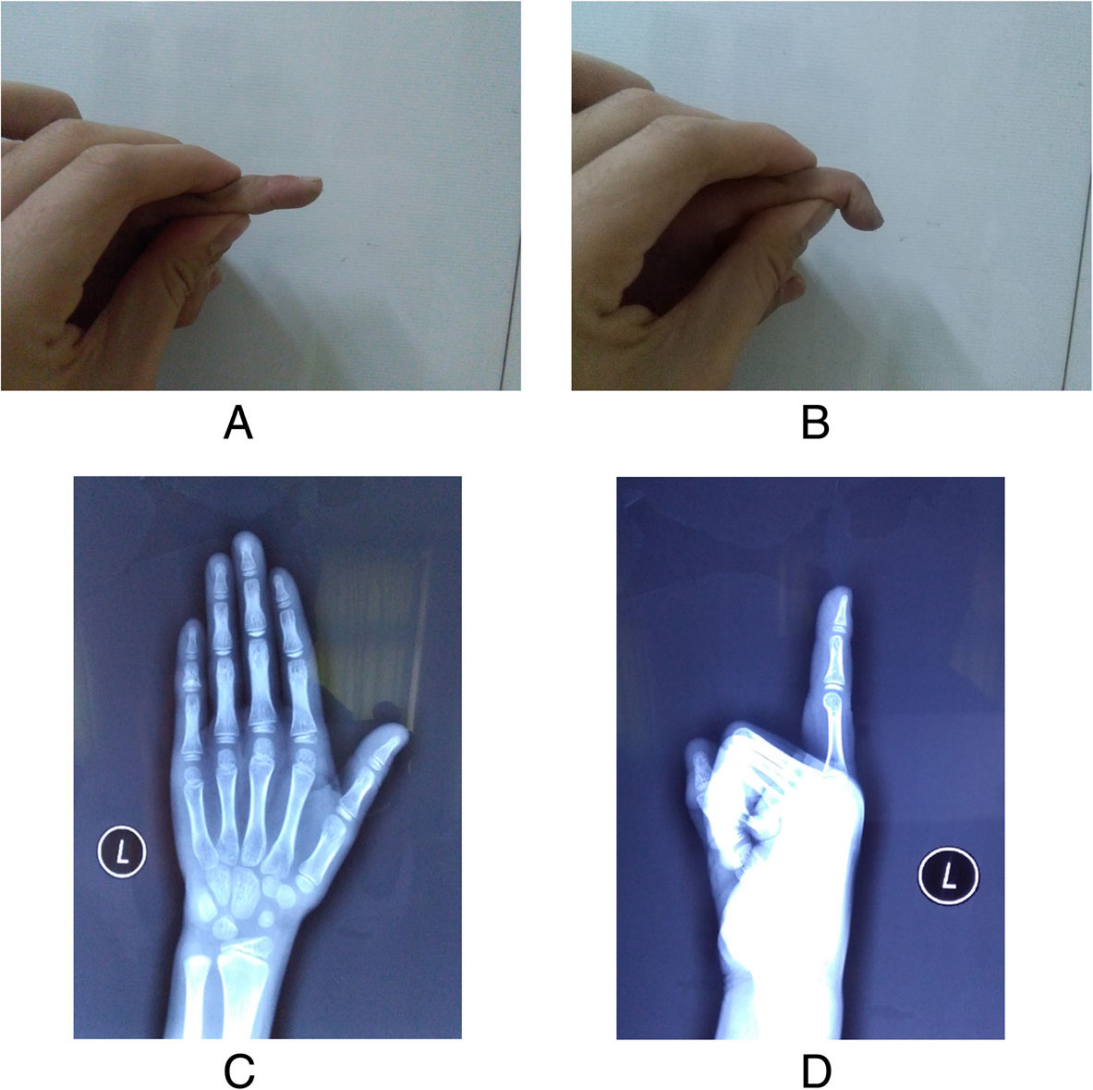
Case 6. a and b, Photographic view taken at 3.8 years postoperatively, showing the range of DIPJ motion. c and d, Anteroposterior and lateral radiograph of the injured finger showed no bone dysplasia, and distal phalangeal physis was normal
